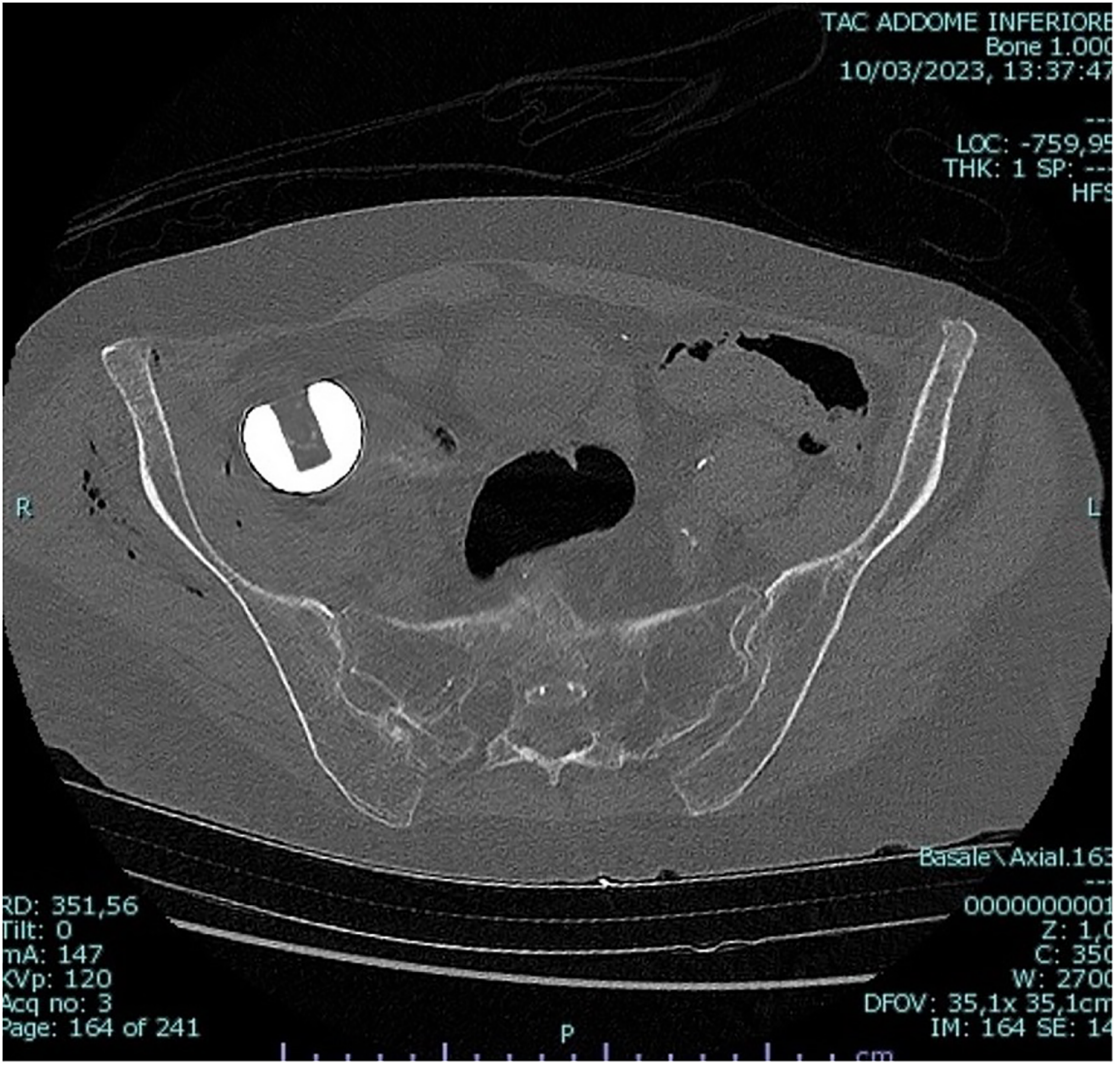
Axial view of the pelvic CT scan.
